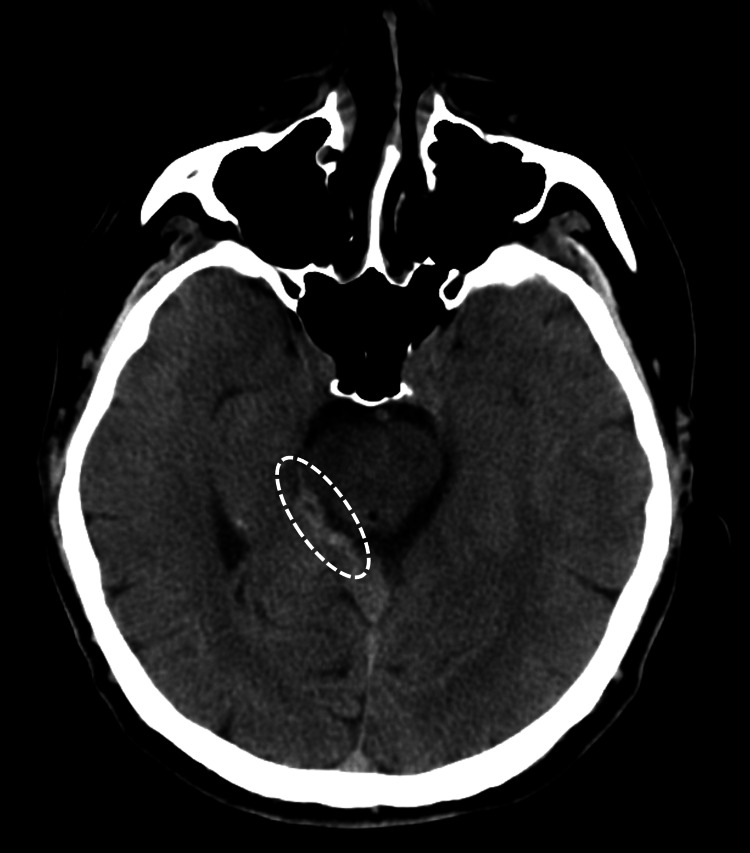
Brain CT of traumatic subarachnoid hemorrhage. Hematoma located in the right ambient cistern, measuring approximately 30 mm × 3 mm (white oval with broken line).
